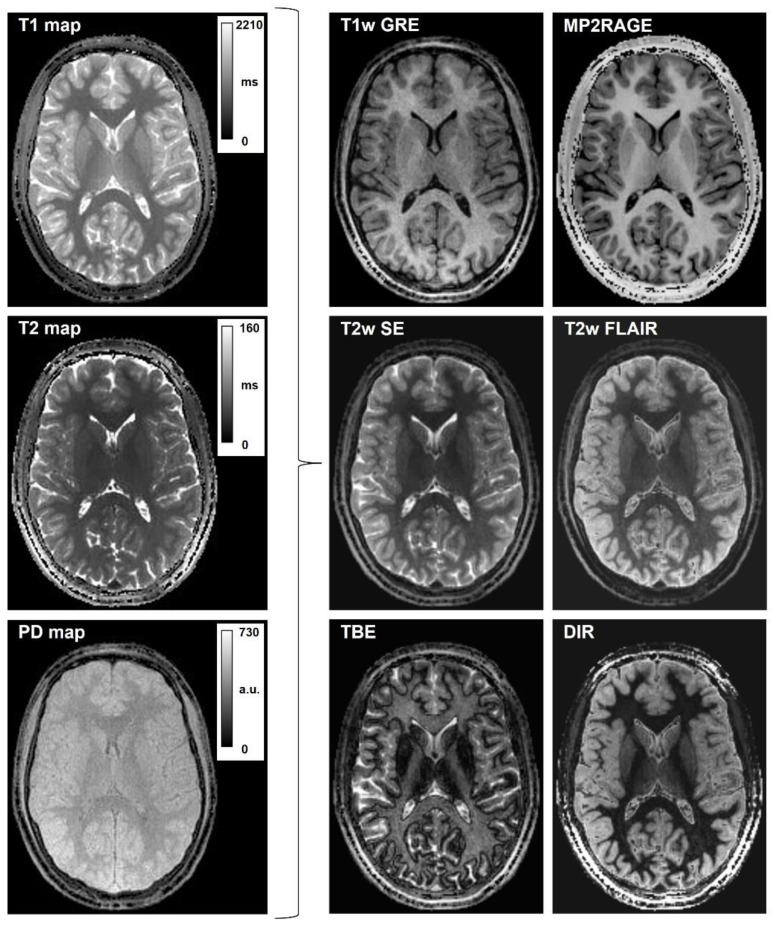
Synthetic T1-weighted and T2-weighted contrasts generated with PySynthMRI. On the left, the input quantitative maps; on the right, the synthesized images.
